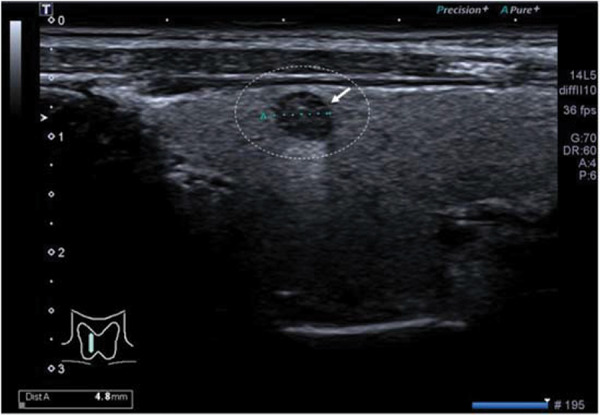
Grayscale ultrasound image of the right thyroid lobe of a 35-year-old female patient. The white circle and white arrow indicate a suspicious thyroid nodule. The maximum diameter of the nodule in the transverse view was 4.8 mm.
